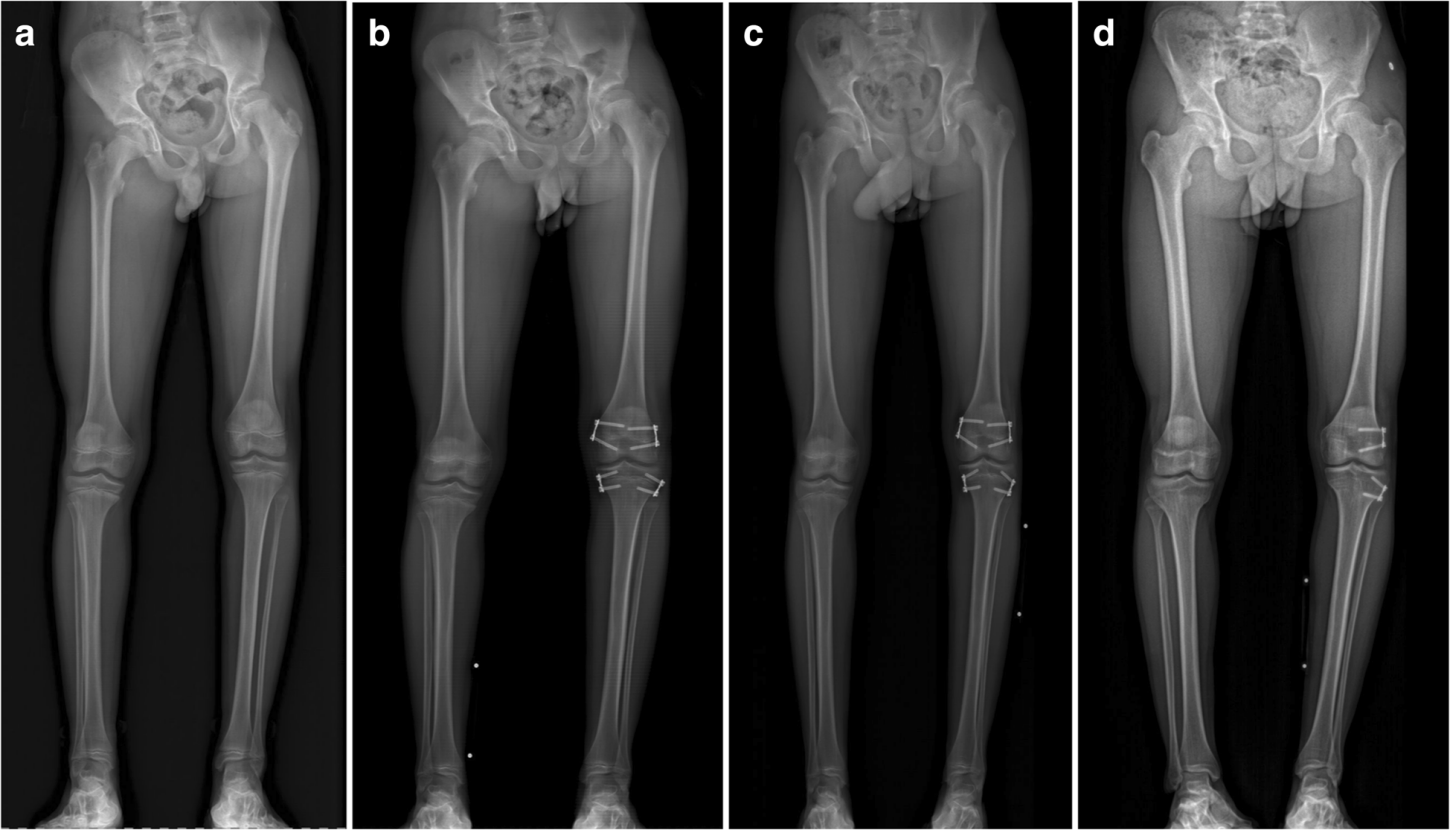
Case of a 14-year-old male patient with idiopathic limb length discrepancy. a Preoperative X-ray, demonstrating pelvic obliquity and shortening of the right leg of 3.9 cm. b Postoperative X-ray after the patient was treated with tension band plates on both medial and lateral epiphyses of the distal femur and proximal tibia. c At 1-year follow-up, partial metal removal had to be performed due to the progression of varus malalignment. Tension band plates of the medial distal femur and medial proximal tibia were removed. d At 2.5-year follow-up, varus malalignment was improved to 6° with a residual LLD of 1.4 cm
